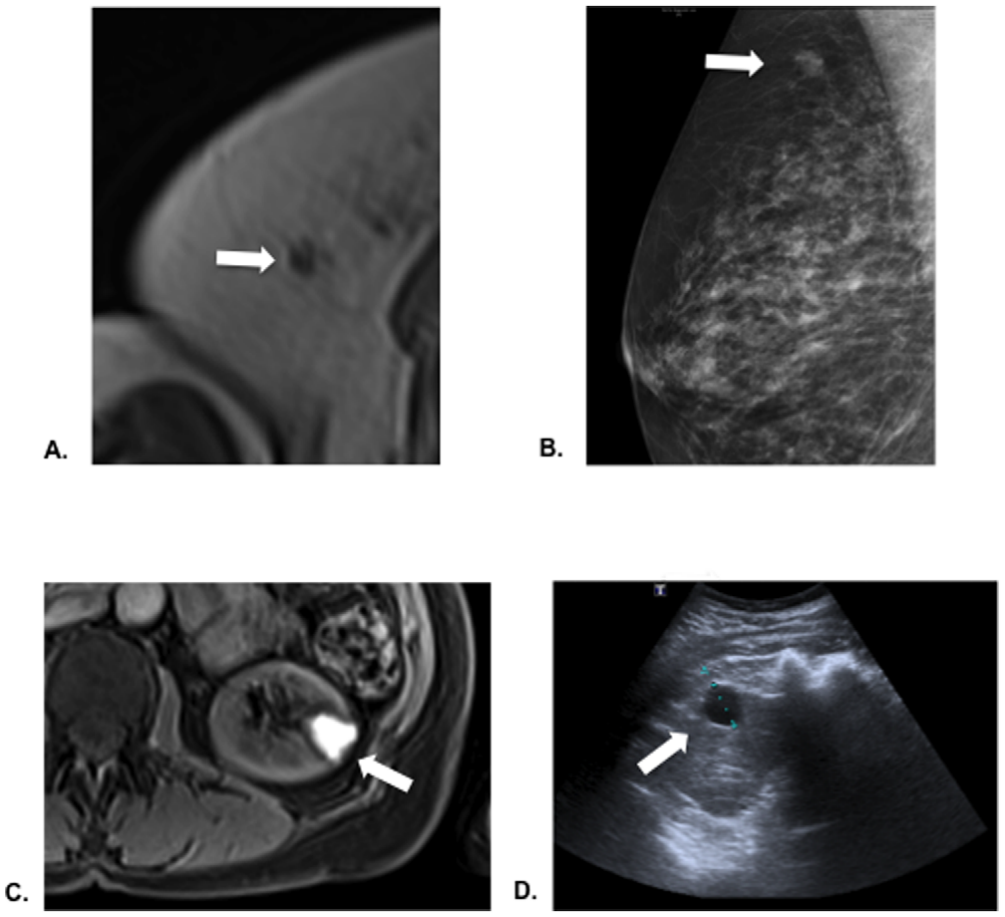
Examples of two subjects with incidental findings from research MRI scans and their associated follow up investigations. Panel A shows an in-phase axial Dixon VIBE MR image displaying a lesion (white arrow) in the right breast classified as being of high clinical significance. Panel B shows the clinical mammogram image that correlate to A. In Panel C a cystic lesion arising from the upper pole of the right kidney (white arrow) is seen on a water-only Dixon VIBE MR image and classified as being of low clinical significance. This was further diagnosed as a cortical kidney cyst (Bosniak Type I) on follow up ultrasound scan (Panel D).
